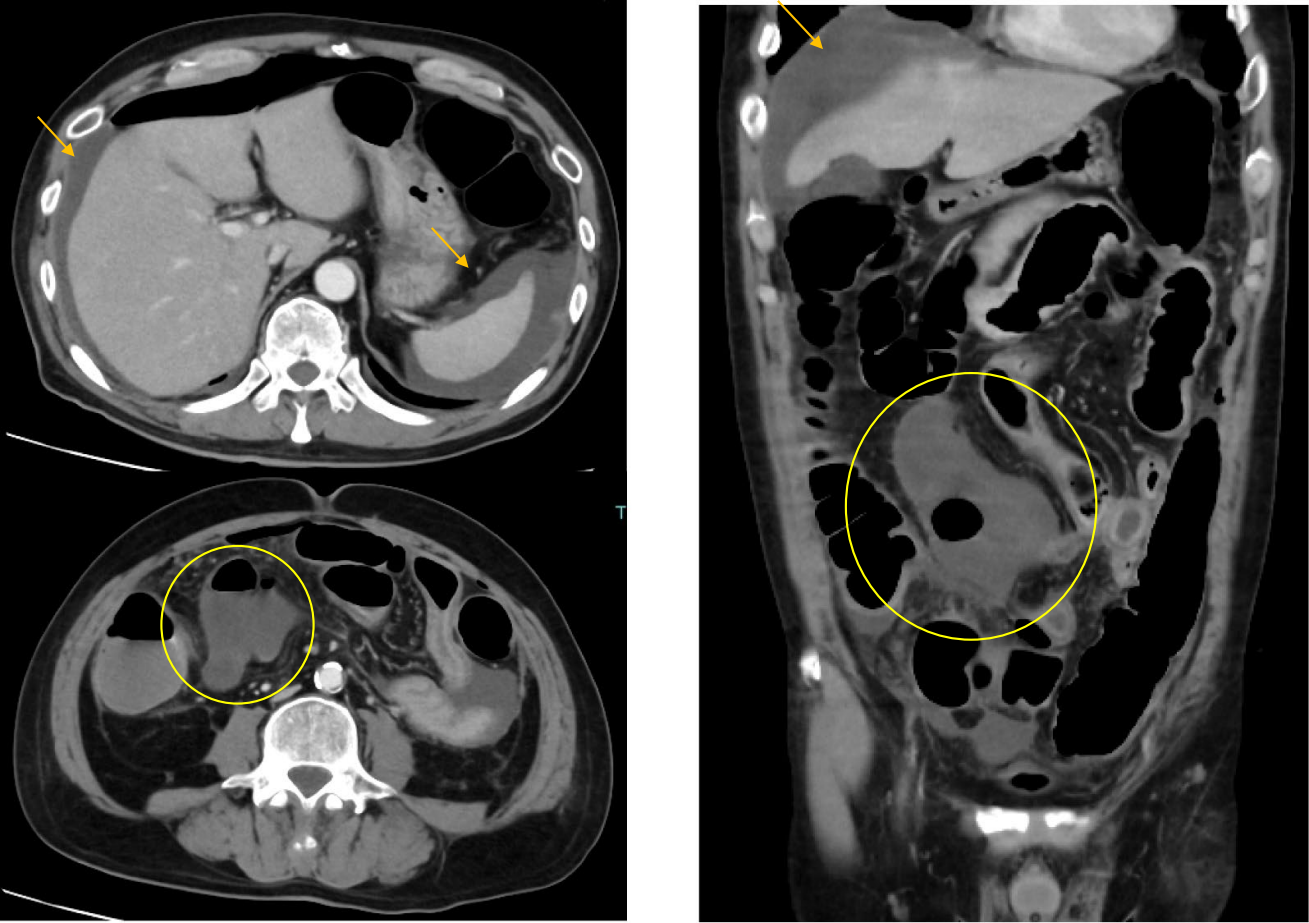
Abdominal contrast-enhanced CT findings. (10th day after hospitalization): Ileum ischemia(○) and ascites ( →)were observed
